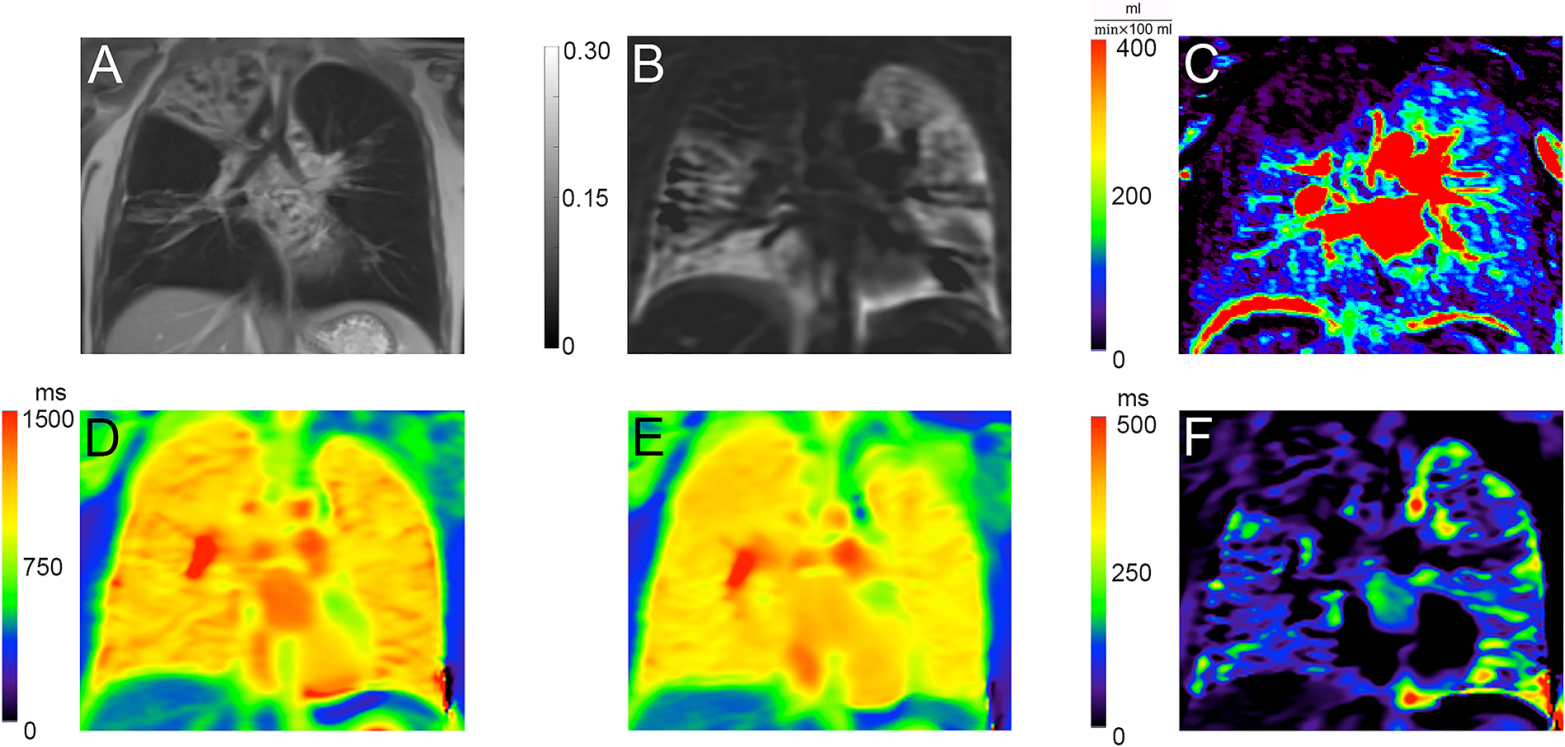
Incidentally discovered consolidation in the right upper lobe in a CF patient. A: T2-weighted; B: Fractional ventilation map; C: Calculated perfusion map; D: Room air T1 map; E: Oxygen enhanced T1 map; F: Delta T1 map.
